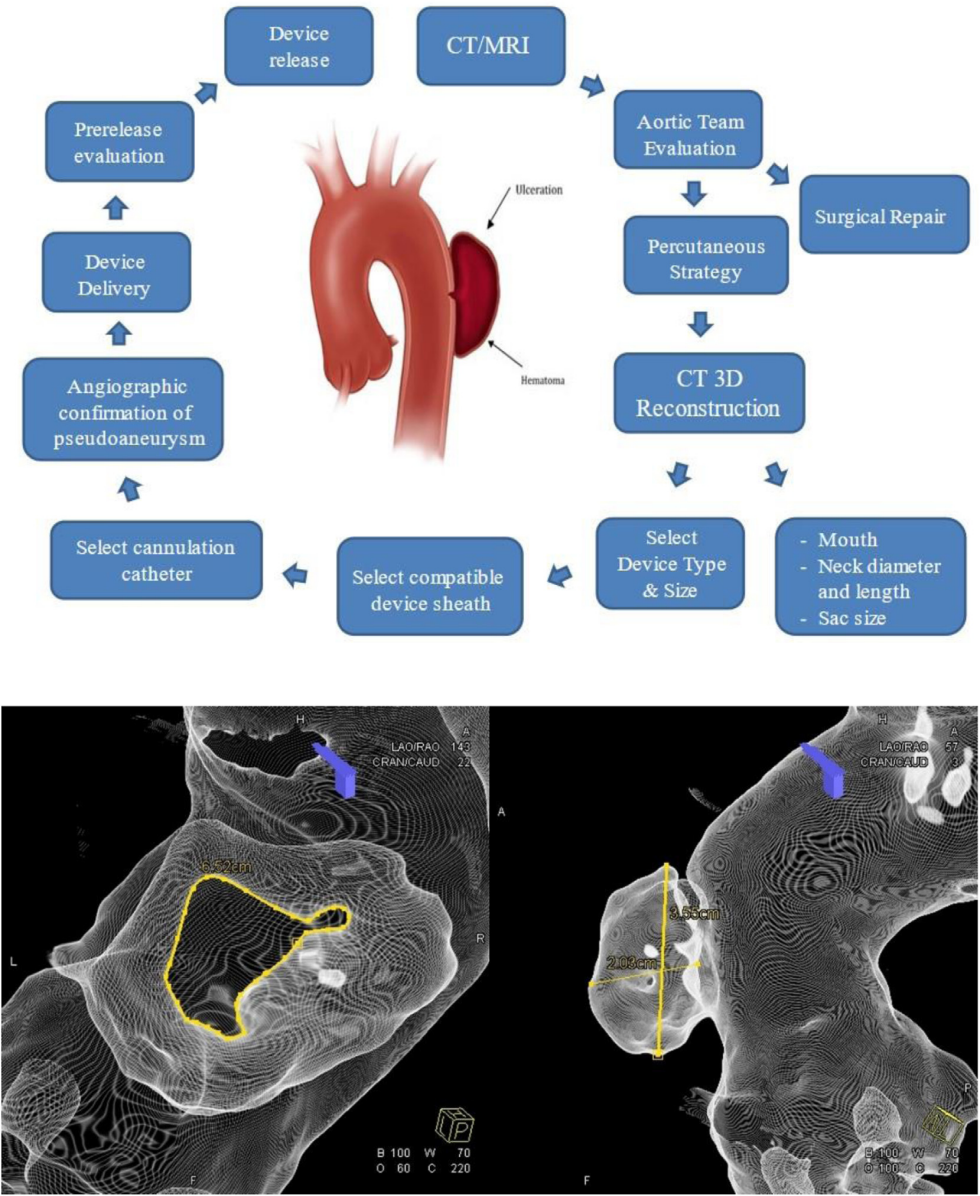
Our institution's procedural approach and some imaging techniques used for the percutaneous aortic pseudoaneurysm (AP) repair. The flowsheet on top outlines our procedural approach steps to percutaneous AP repair. The 2 images at the bottom show an example of our utility of 3-dimensional computed tomography (3D CT) reconstruction in measuring the AP mouth perimeter (bottom left) and AP sac dimensions (bottom right). MRI, magnetic resonance imaging.
